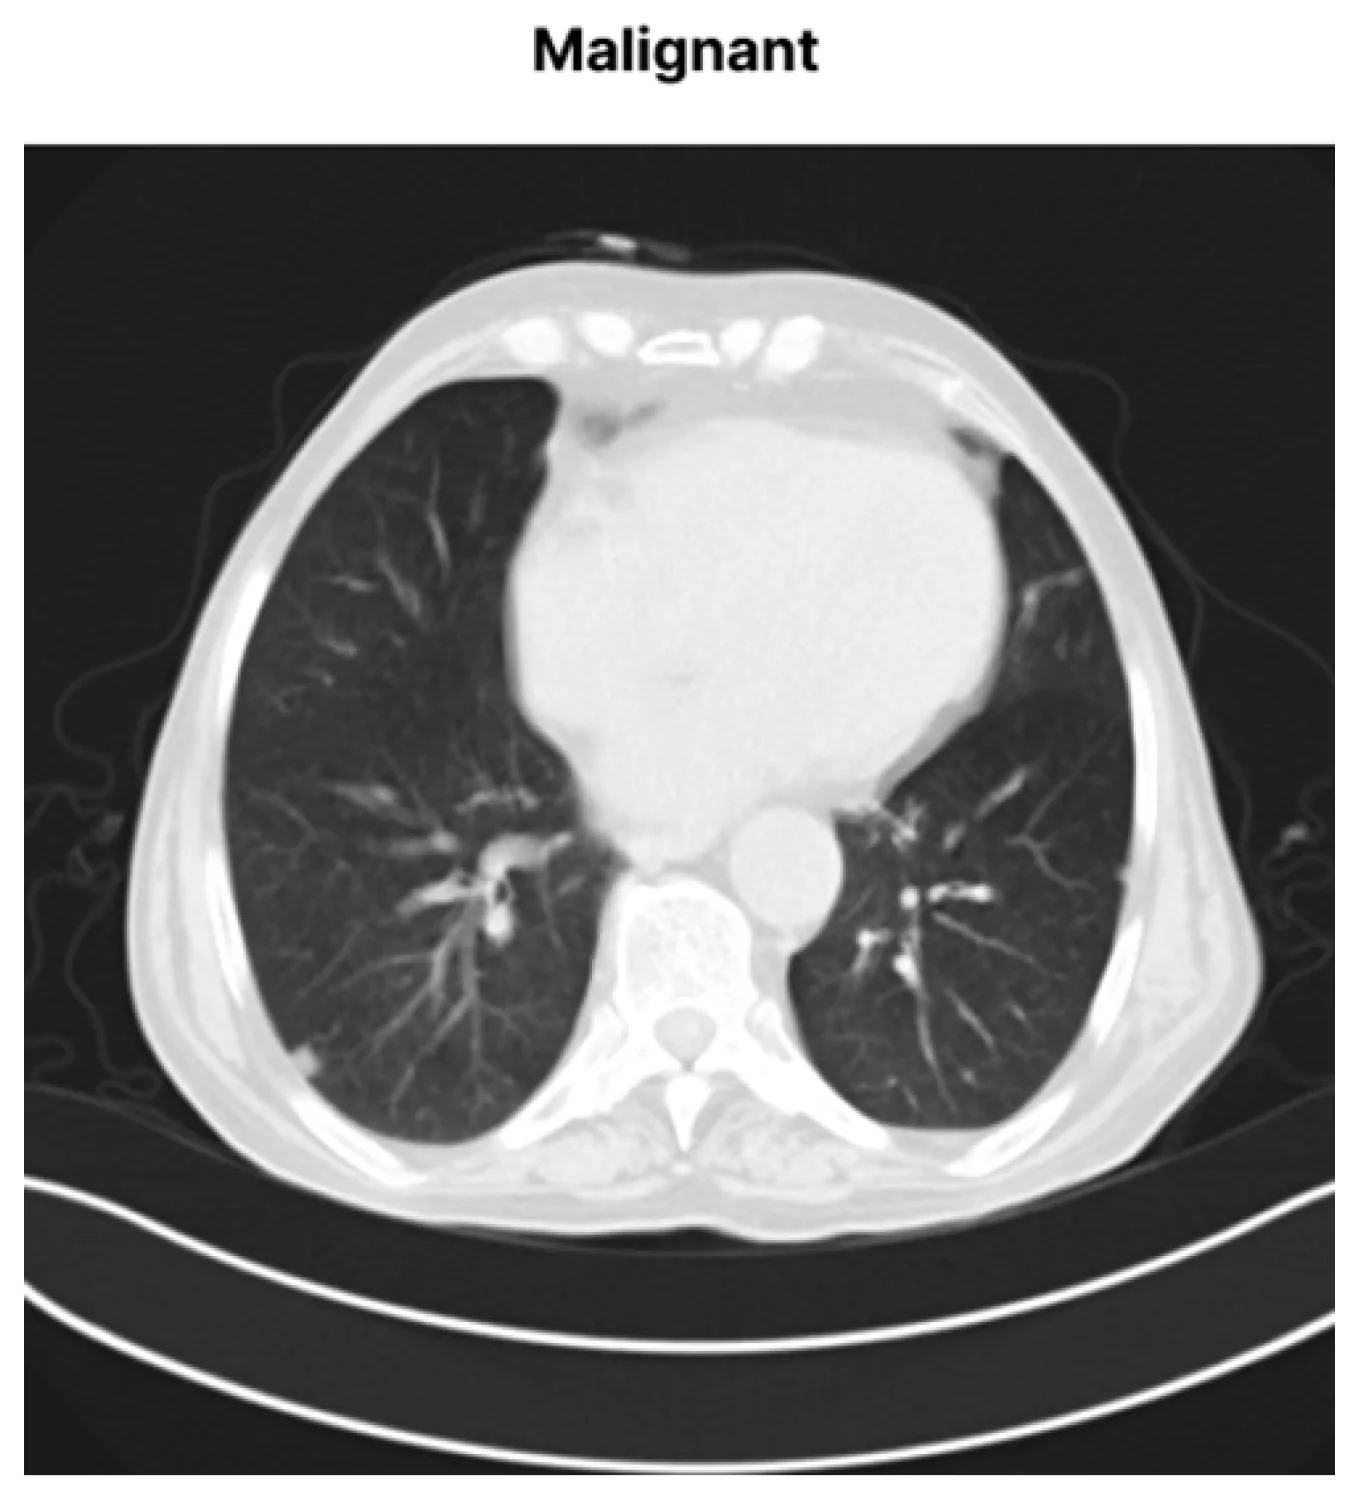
Malignant CT scan image sample.
